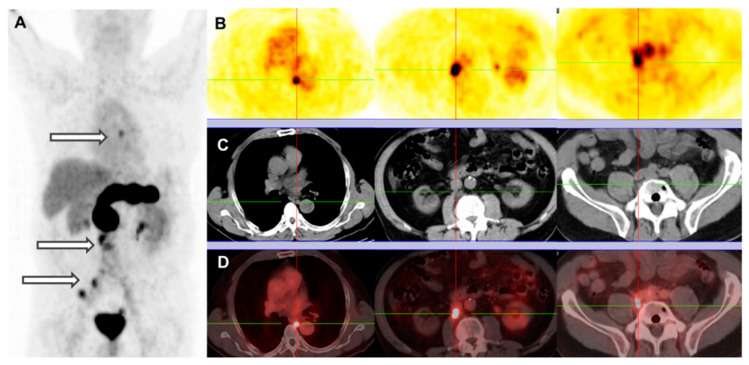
Patient with PC, post radical prostatovesiculectomy with pelvic lymphadenectomy, IMRT, and androgen deprivation therapy (PSA on date of scan, 21.77 ng/mL). [^68^Ga]Ga-NeoBOMB1 PET/CT: (A) PET MIP; (B) serial PET transverse; (C) corresponding CT transverse; (D) fusion PET/CT images. Multiple mediastinal, abdominal, paraoesophageal and pelvic lymph node metastatses (arrows and crossbars). This research was originally published in *JNM* 2017 [34].
